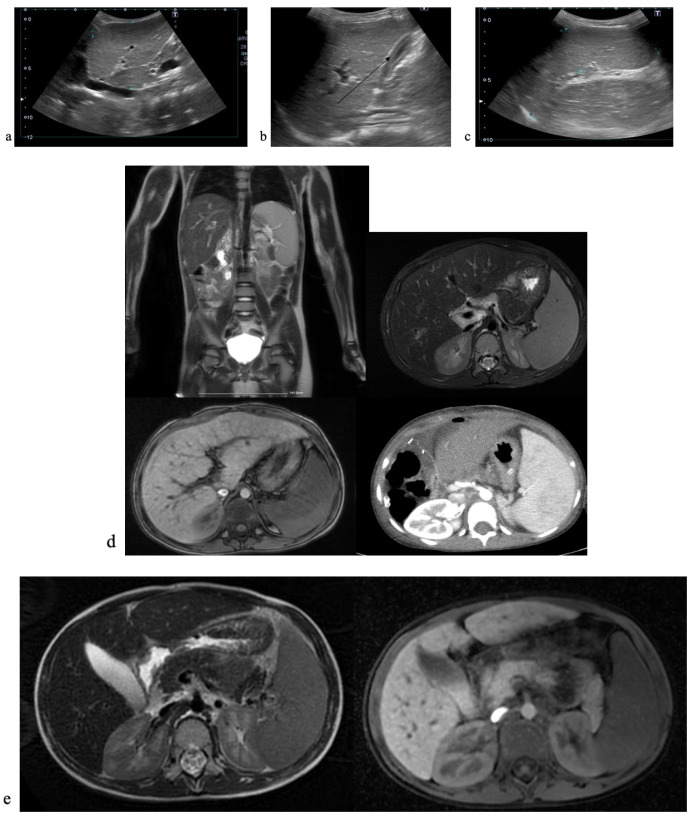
Imagistic findings in case 2. (a) Abdominal US—liver nodules. (b) Abdominal US—thick-walled, double-layered gallbladder. (c) Abdominal US—enlarged spleen. (d) Abdominal MRI—enlarged liver and spleen. (e) Abdominal MRI—thick-walled, double-layered gallbladder.
